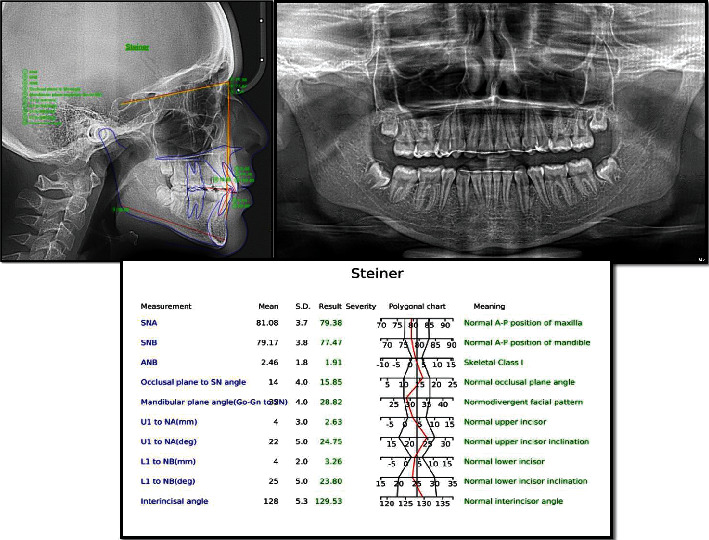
Posttreatment cephalogram and panoramic radiograph with analysis of the lateral cephalogram using the Steiner analysis.
